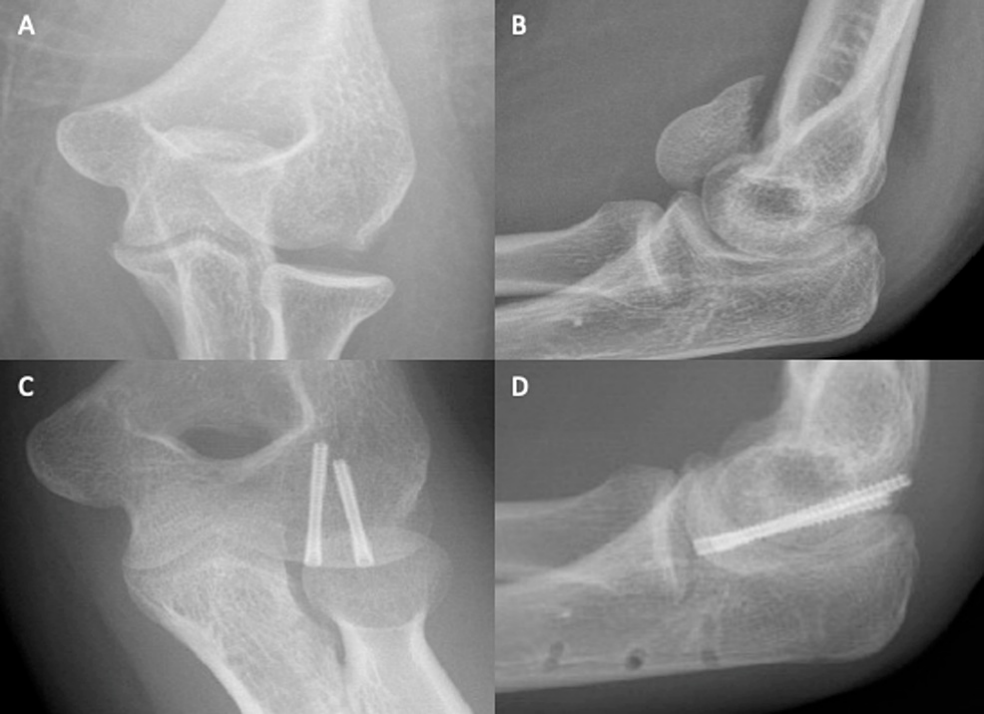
Plain elbow X-rays of case 6. Figure 8A, 8B shows anteroposterior and lateral views, respectively, of a type I capitellum fracture. Figure 8C, 8D shows anteroposterior and lateral views, respectively, of the elbow after ORIF. ORIF: open reduction and internal fixation
